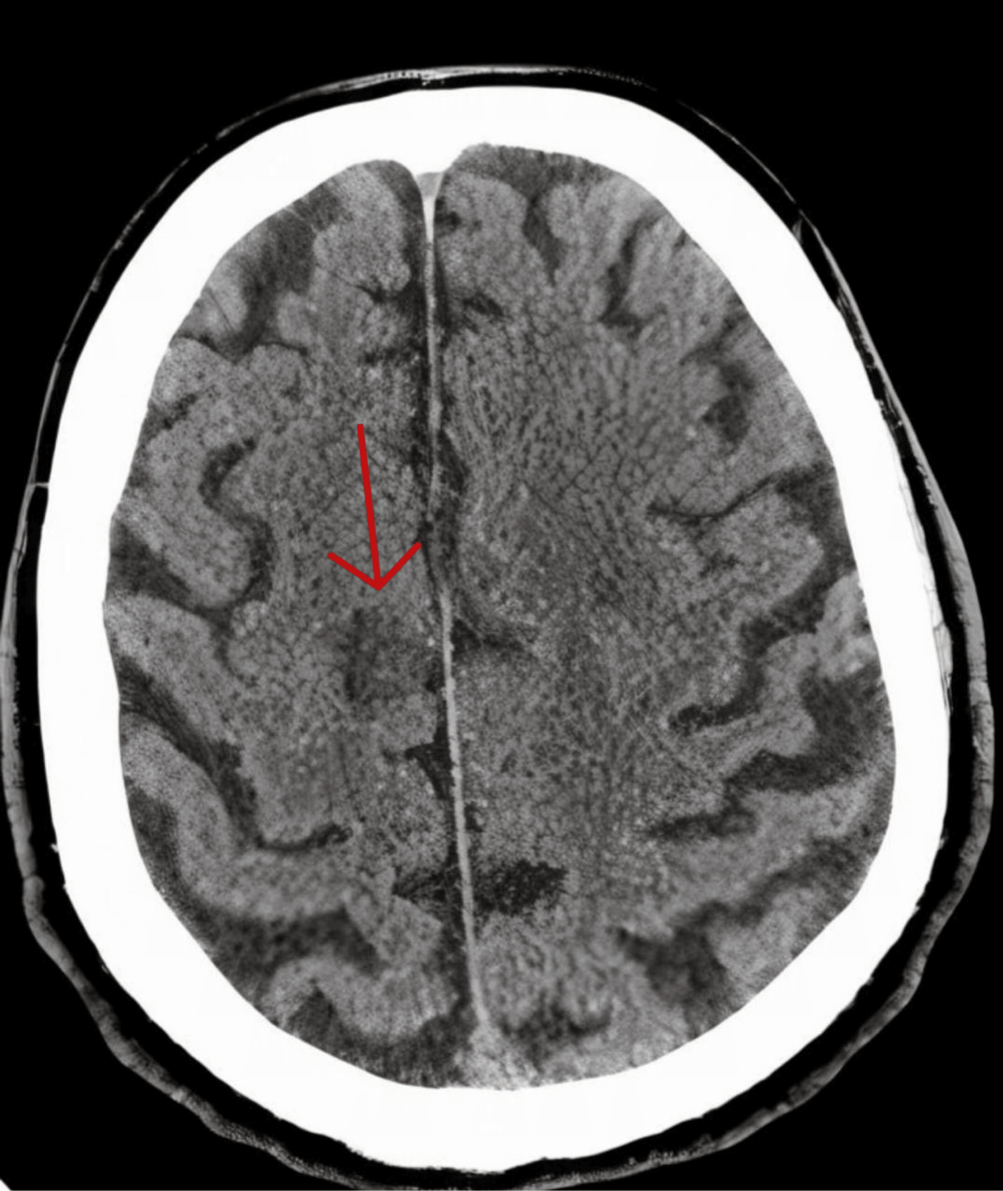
Axial non-contrast computed tomography of the brain Right frontal parasagittal hypodensity, likely vascular in nature, represents a subacute/chronic ischemic insult with chronic microangiopathic disease.
